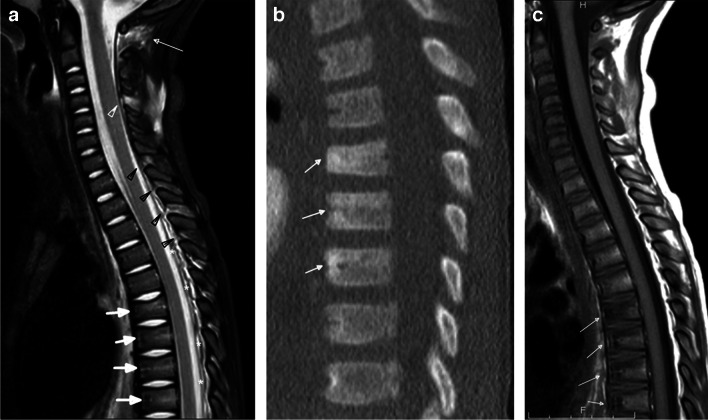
Two-year-old boy presenting with difficulty walking and unexplained bruising. Sagittal STIR MR image (a) of the upper spine demonstrates atlanto-axial ligamentous injury (*thin white arrow*) and interspinous ligamentous edema, widening of the interspinous distance, and disruption of the ligament flavum at C4-C5 (*white arrowhead*). There are also multilevel upper thoracic interspinous ligament edema with ligamentum flavum disruption (*black arrowheads*) and epidural fluid in the dorsal aspect of the mid thoracic spine (*white asterisks*). Note biconcave appearance of thoracic vertebral bodies with mild edema signal suggesting subtle compression fractures (*thick arrows*). Sagittal bone algorithm CT image (b) of the thoracic spine demonstrates loss of vertebral body height and sclerosis anteriorly of the T6, T7, and T8 vertebral bodies (*arrows*) with anterior wedging and T1-hypointense signal on the sagittal T1-weighted MR image (c) of the spine (*arrows*)
